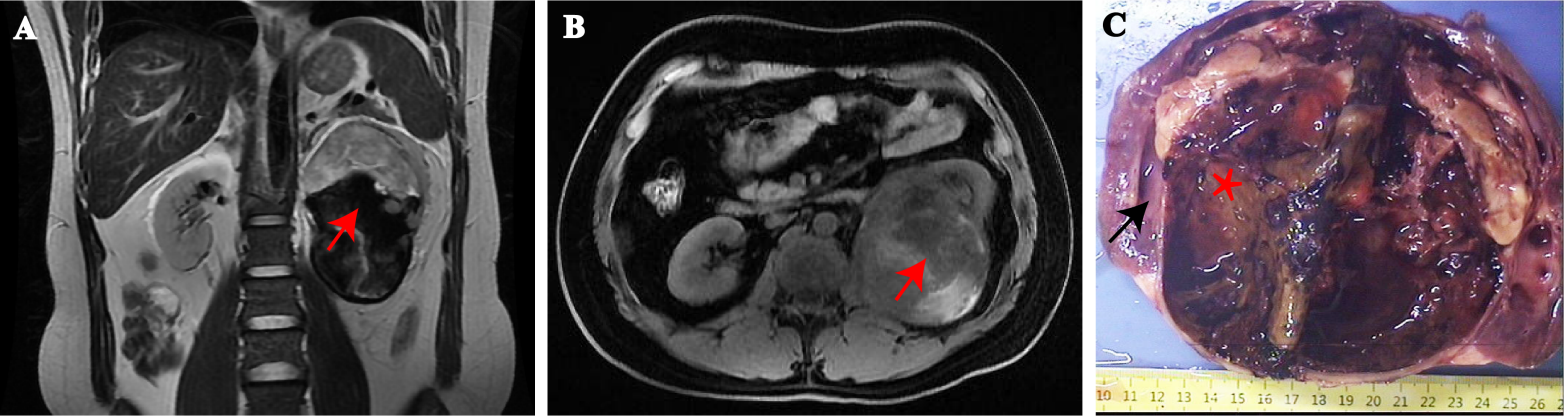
MRI showing a large tumor arising from the hilum of the left kidney. (A) Sagittal and (B) transverse view. (red arrows = tumor). Gross findings, the mass was located at the renal hilum and clearly demarcated from the surrounding renal tissue (C). (black arrows = renal tissue, asterisk = tumor).
